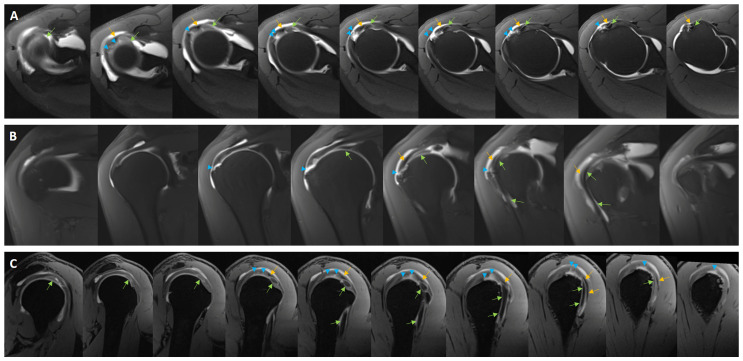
Consecutive axial (A), oblique coronal (B) and oblique sagittal (C) T1-weighted MR arthrograms reveal association between AEST and rotator cuff tear. The consecutive images show partial full thickness tear of the supraspinatus tendon without tear of the subscapularis tendon and AEST. Blue arrowheads = supraspinatus tendon tear; yellow arrows = AEST; green arrows = LHBT.
